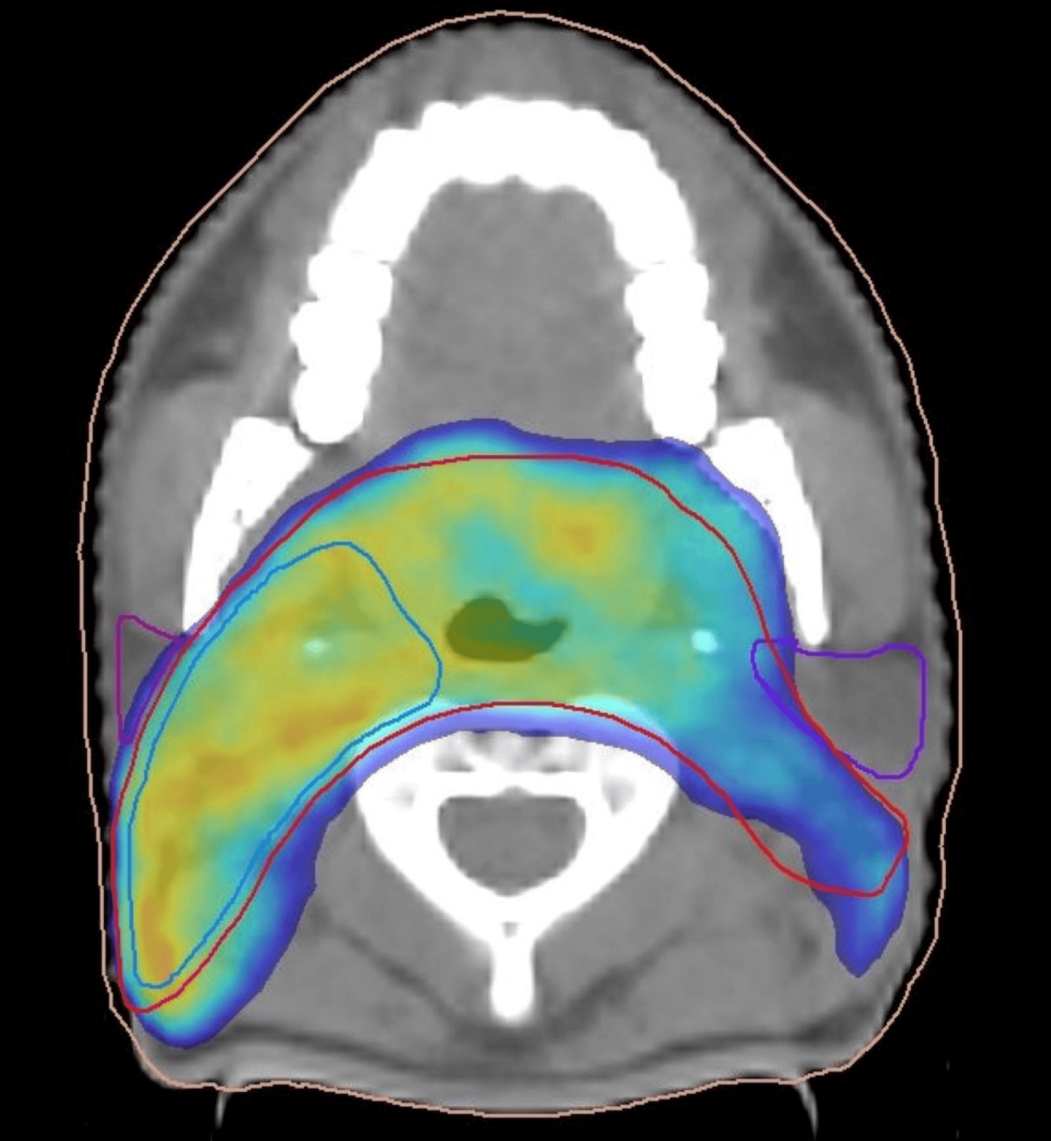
Axial CT slice of a head and neck intensity modulated radiotherapy (IMRT) plan. The dose distribution shows conformal coverage of the primary tumor and nodal planning target volume (PTVs) with sparing adjacent organs at risk.
